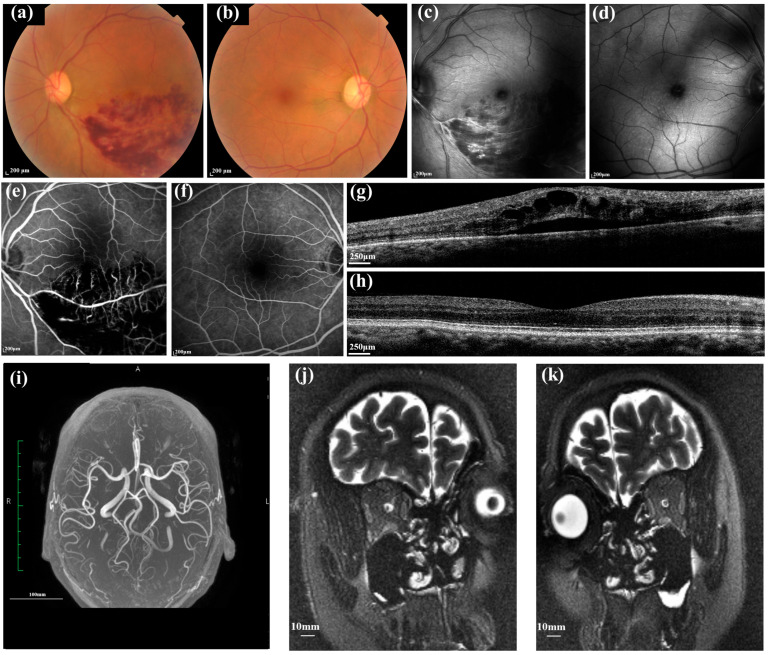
Advanced multimodal images of a 62-year-old female with BRVO in the left and normal contralateral right eyes. The best corrected visual acuity was 20/70 in the left eye and 20/30 in the right eye. Intraocular pressure was 18 mmHg in both eyes. Color fundus photos showed diffuse hemorrhages in the inferior temporal quadrant in the left eye (a), and the right eye looks normal (b). Infrared images of the left (c) and right eye (d). A late-phase frame of the fluorescein angiogram demonstrates dilated, tortuous veins with the area of fluorescein blockage in the left eye (e); the right eye looks normal (f). OCT image of the left eye shows the intra- and sub-retinal fluid and hyper-reflective foci in the inner retina (g), and the macular structure in the right eye is normal (h). The MRI imaging showed a reduced number of the middle cerebral artery and its branches in the affected left eye (i). The ONSASW behind the bilateral globe showed no difference using the MRI ((j,k); oblique coronal T2WI-FRFSE image with fat suppression). The advanced stage of BRVO, complicated by macular edema, showed that the retinal vessel was intensively affected, which correlates with impaired cerebral vessels. This case also showed that the ONSASW did not significantly differ from the contralateral eye, further supporting the hypothesis that BRVO may have a close relationship with cerebrovascular events, not glaucoma. BRVO: Branch retinal vein occlusion. ONSASW: Optic nerve subarachnoid space width. OCT: Optical coherence tomography.
